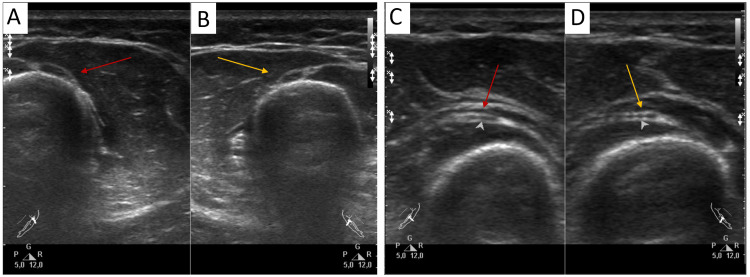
The presented transverse sonograms depict the affected (left, red arrow) and unaffected (right, orange arrow) sides of the radial nerve at the spiral groove (A, B) and the deep branch of the radial nerve at the supinator muscle (C, D). A high-resolution ultrasound examination revealed a mild enlargement in the cross-sectional area of the radial nerve on the affected side at the spiral groove only (A), compared to the contralateral side
